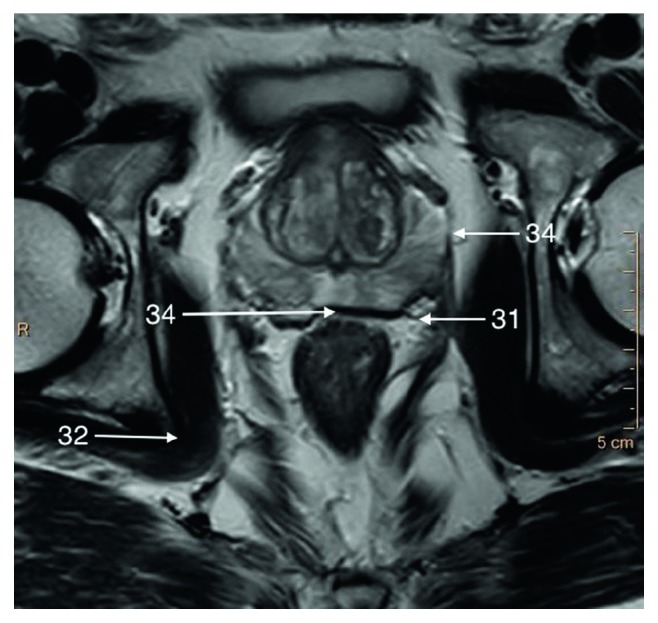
T2-weighted axial image of a middle part of the prostate gland.
